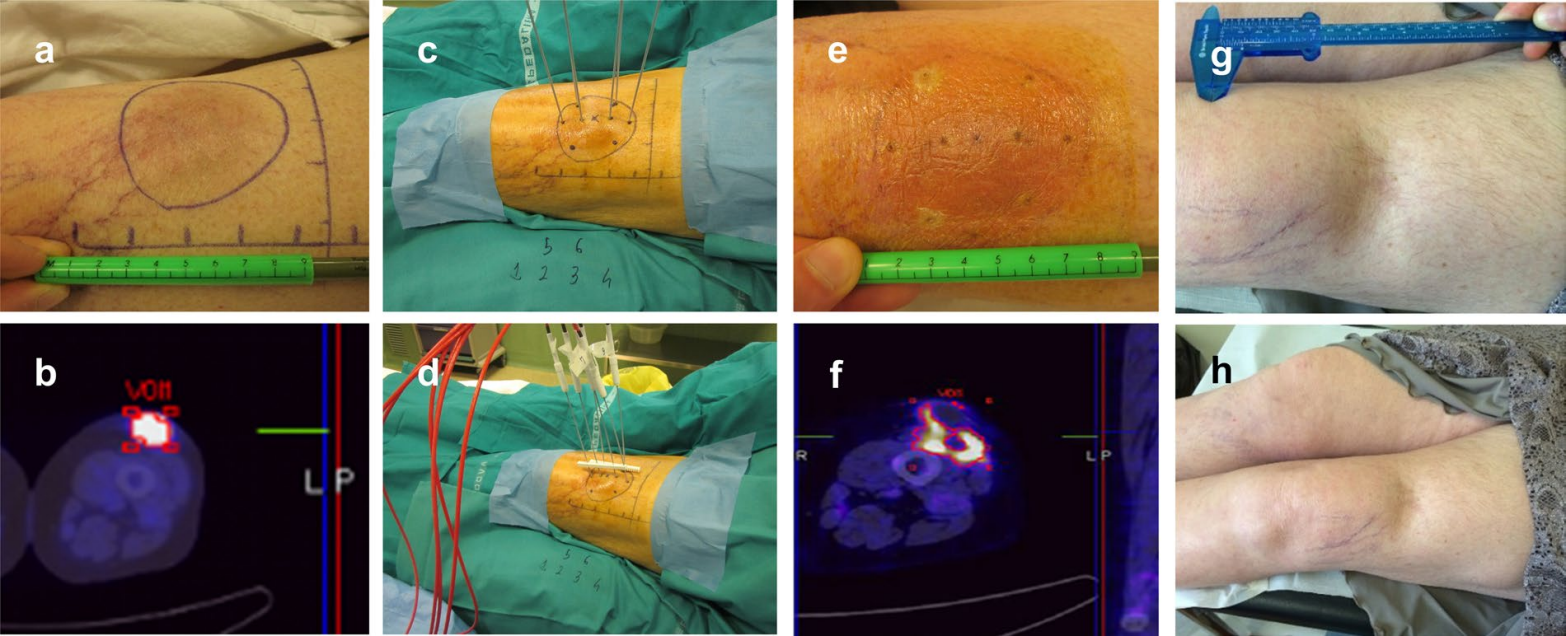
Tumour response in a melanoma patient. This woman presented with a 4-cm soft tissue metastasis in the anterior aspect of the thigh following previous systemic treatment and bilateral adrenalectomy for metastatic disease. (a) Baseline clinical presentation and (b) PET-CT scan. (c) Intraoperative electrode insertion (the treatment plan included two separate electrode placements to encompass the target volume). (d) Connection of the electrode probes to the pulse generator. (e) Early post-treatment erythema. (f) One-month PET-CT scan showing marked metabolic response and residual inflammatory features at the border of the ablation zone. (g) Six-month and (h) 2-year clinical follow-up.
